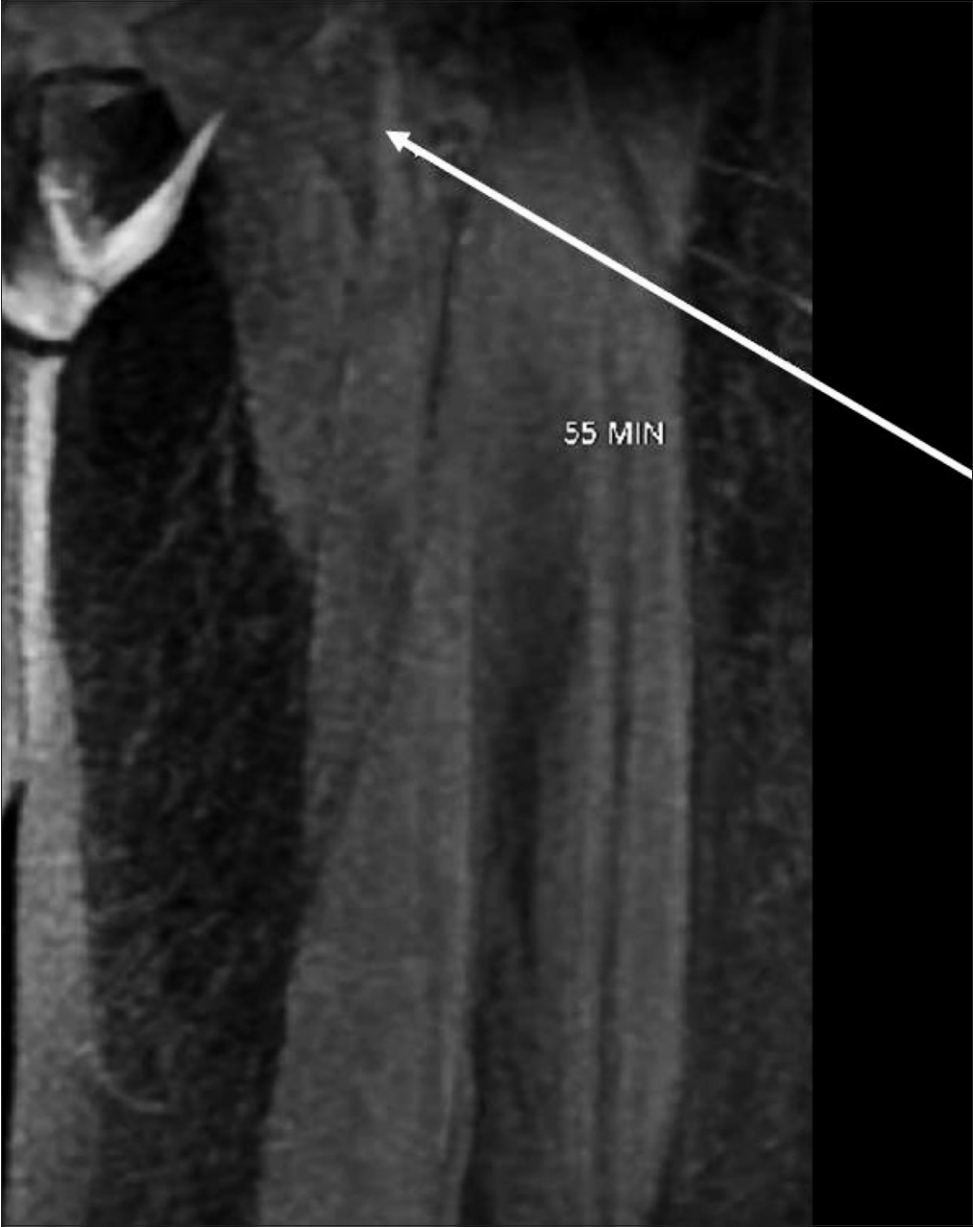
3D spoiled gradient-echo MRI image obtained at 55 min shows dilated lymphatics (arrow) reaching upto the groin
